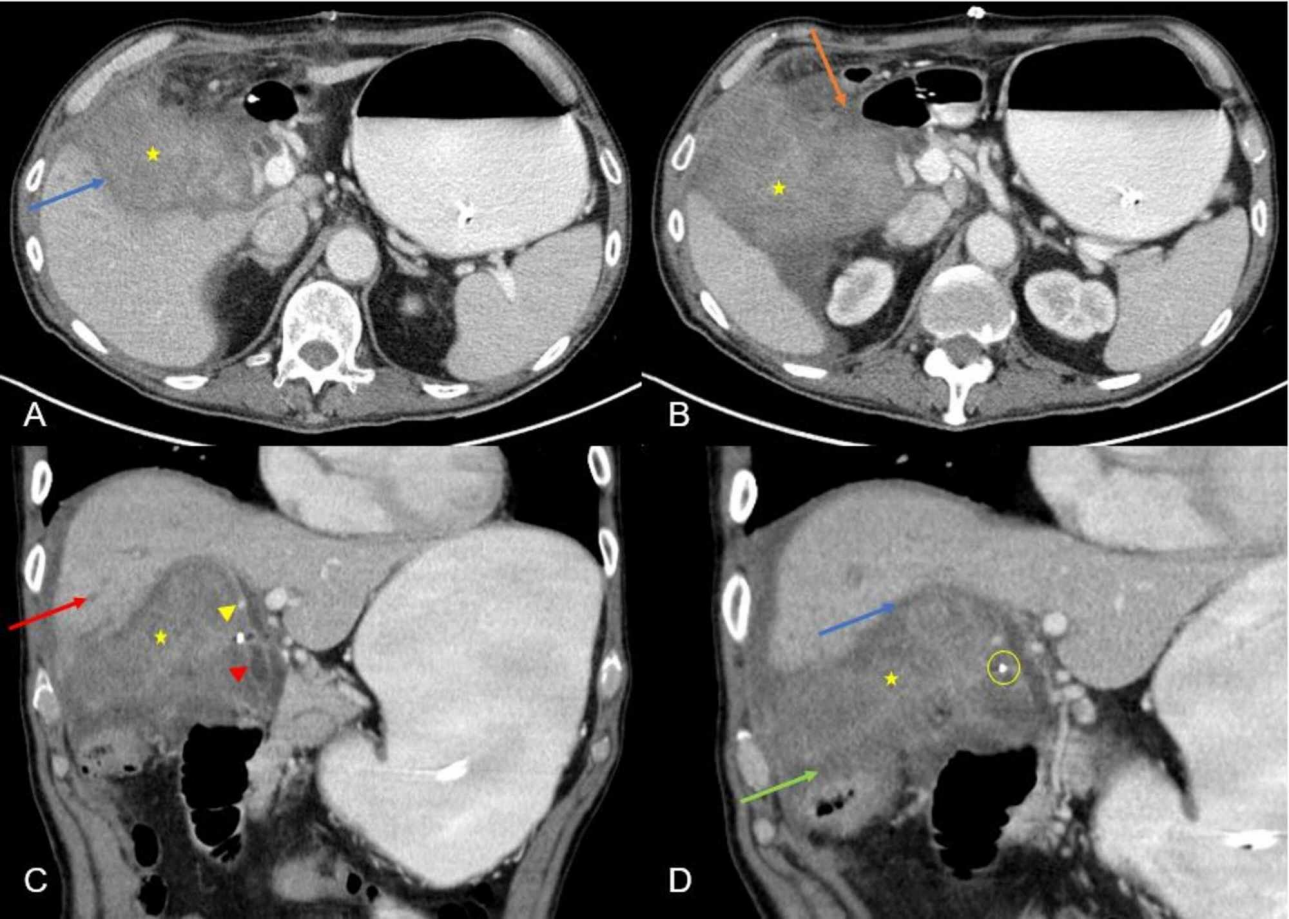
Interval increase in size of the mass. Multiple abdominal CT images: axial images (A, B) and coronal images (C and D) demonstrating interval increase in size of the mass with progressive infiltrative features in the gallbladder fossa (yellow star) resulting in involvement of multiple structures. There is loss of fat plane with the pylorus and proximal duodenum (orange arrow on image B) resulting in gastric dilation. There is loss of fat plane with the undersurface of the liver (blue arrows on image A and D). The mass is showing progressive compression of the CBD, right hepatic duct and inseparable from the cystic duct stump (red arrowhead on image C). There is upstream right intrahepatic biliary dilatation (red arrow on image C). There is encasement of the right hepatic artery at the porta hepatis (yellow arrowhead on image C) with compression of right portal vein. There is loss of fat plane with hepatic flexure (green arrow on image D). Surgical clip (yellow circle on image D) from prior cholecystectomy. CBD, common bile duct
